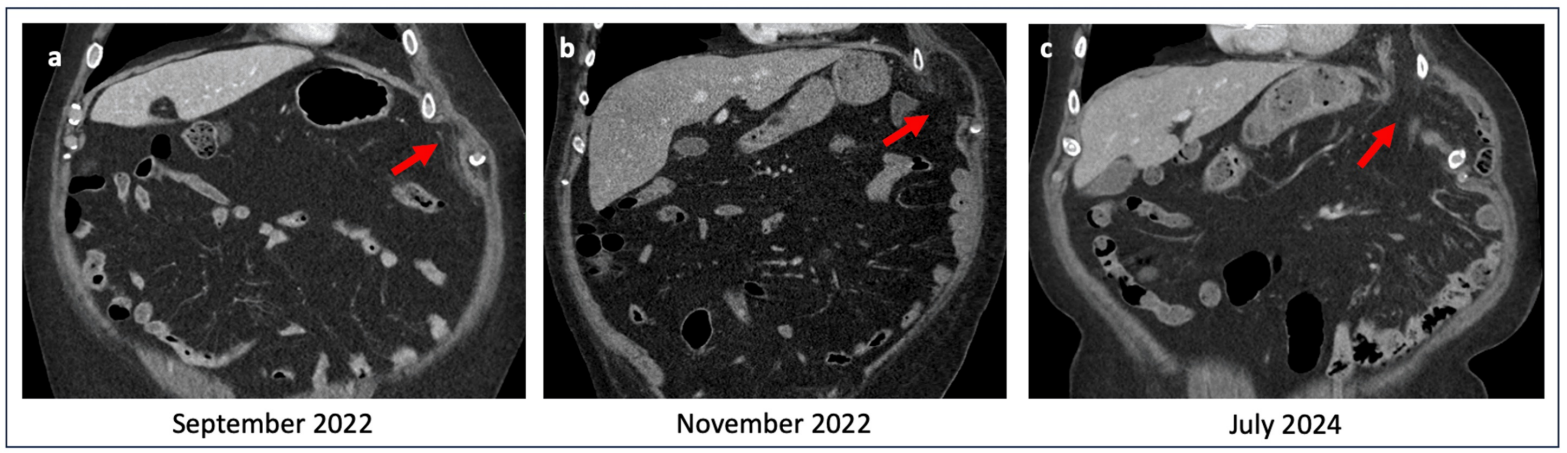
Frontal view of thoracoabdominal CT scan evolution of the left transdiaphragmatic intercostal hernia over time.
